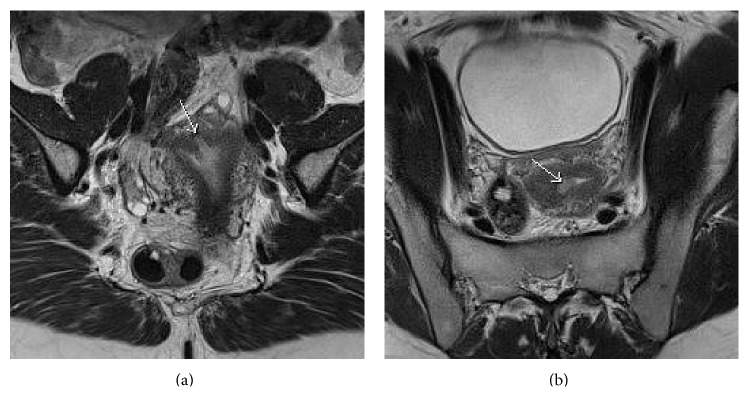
Coronal (a) and axial (b) T2WI MRI shows endometrial mass (white arrow) invading the myometrium.
